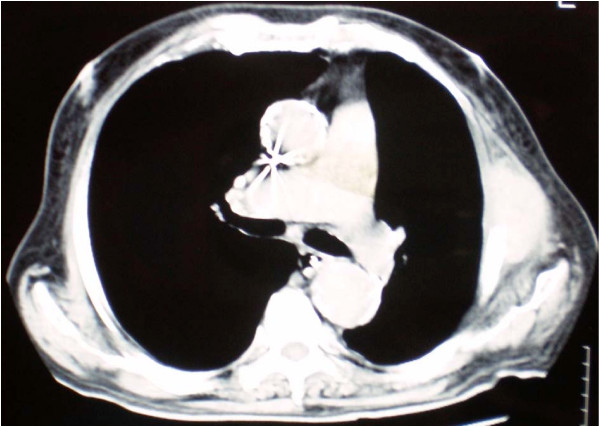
CT scan image of the patient's chest showing osteolytic abnormalities of the 5^th ^left rib, consistent with secondary deposits.
